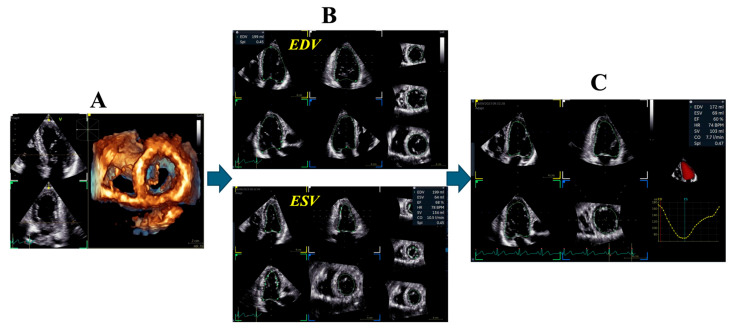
Example of automated calculation of left ventricular (LV) volume and ejection fraction using the three-dimensional viewing in echocardiography. (A) Manual selection of a pyramidal apical four-chamber acquisition by the operator. (B) Identification of two landmarks (the apex and the center of the mitral leaflet coaptation), followed by automated endocardial border delineation in systole and diastole across multiple reconstructed planes. (C) Automated quantification of left ventricular volumes and function (ejection fraction, stroke volume, cardiac output). At this step, the operator has the option to edit and refine the contours.
